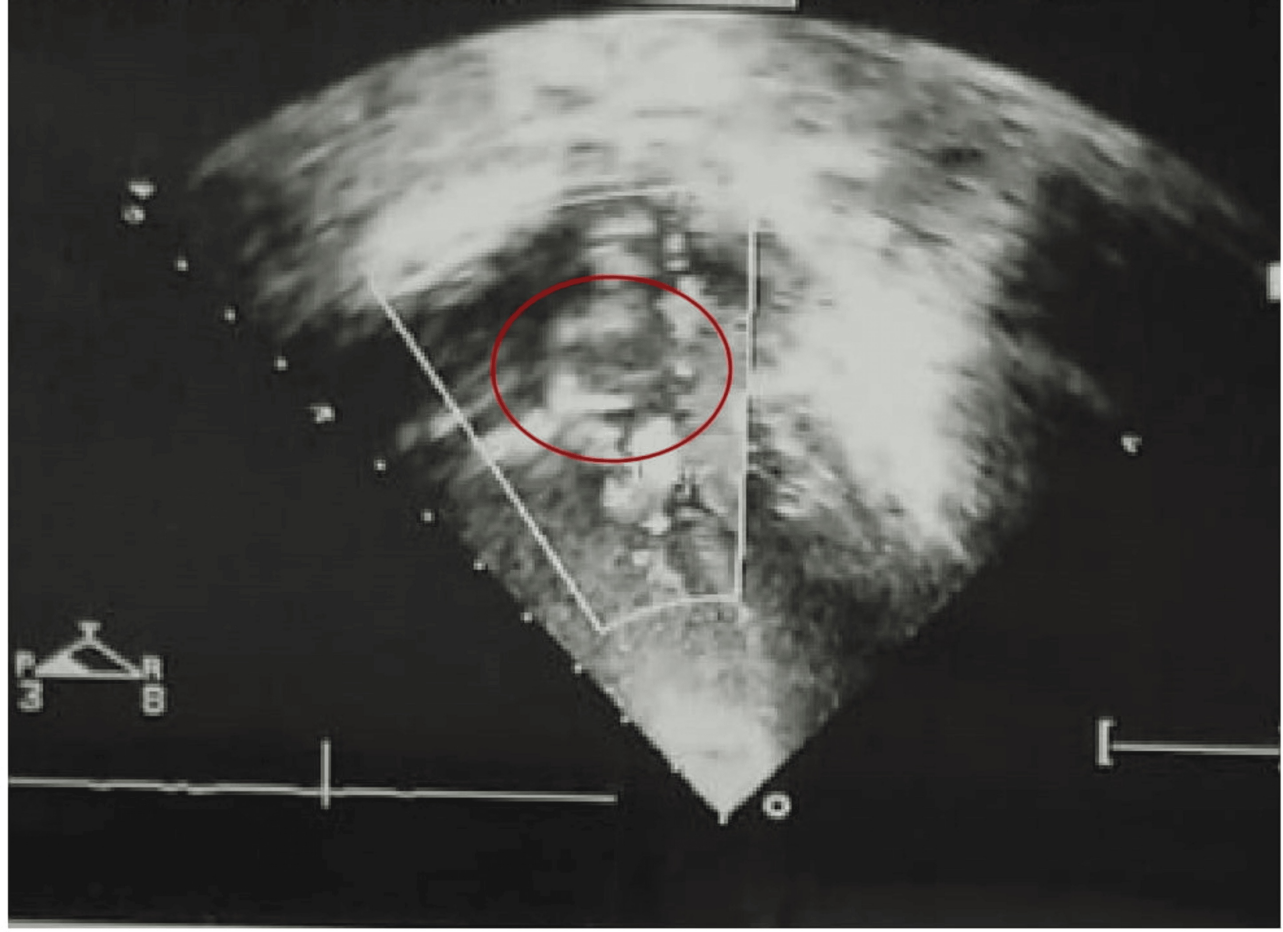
Echocardiography of the patient showing an 8-mm nonrestrictive ventricular septal defect with severe pulmonary hypertension and Eisenmenger syndrome
